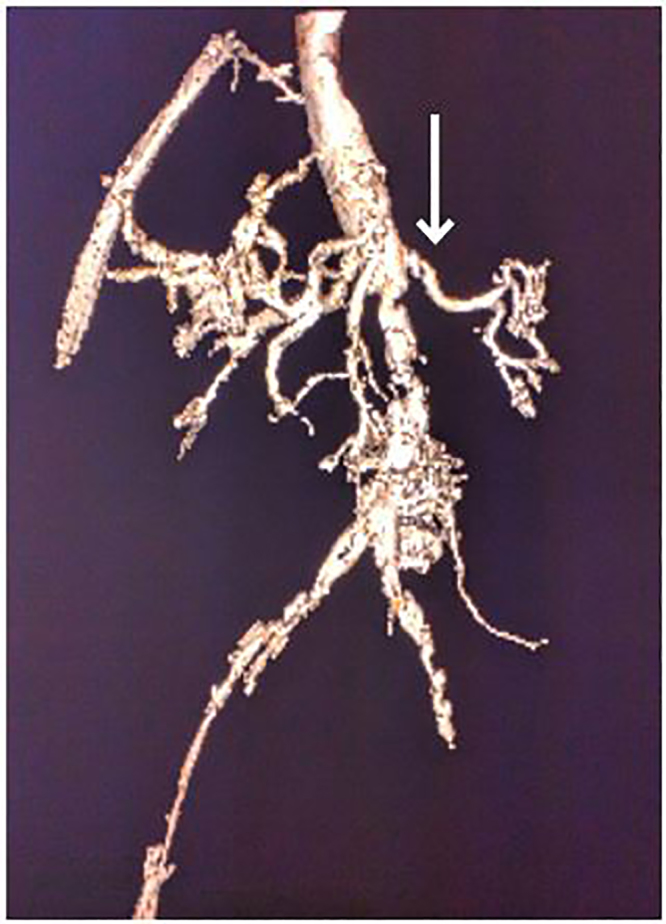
Computed tomography angiography of the abdominal aorta and bilateral lower extremities with runoff in three-dimensional reconstruction. Severe narrowing at the origin of the left renal artery is visible (arrow).
